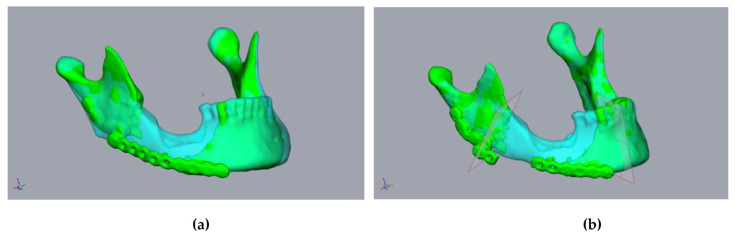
Planning using a CT scan before resectioning: A 55-year-old male patient presented with a fractured alloplastic reconstruction-plate after the partial resectioning of the mandibula due to radio osteonecrosis. (a) The mandibula before resectioning (clear cyan) and the current defect situation (green). (b) The repositioned mandibular stumps and planned resection planes.
